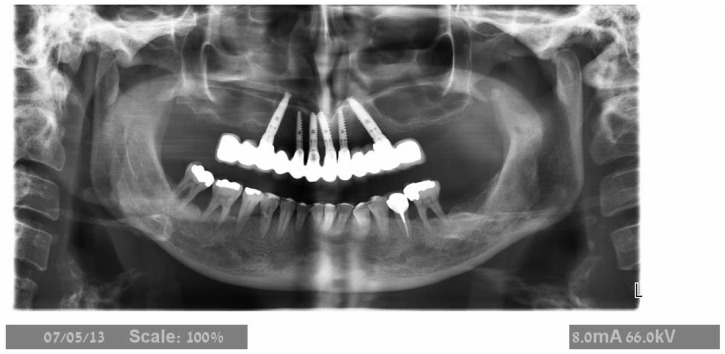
Panoramic X-ray after implant placement with the final prosthesis. The prosthesis was designed with two distal cantilevers on the right side and one on the left.
